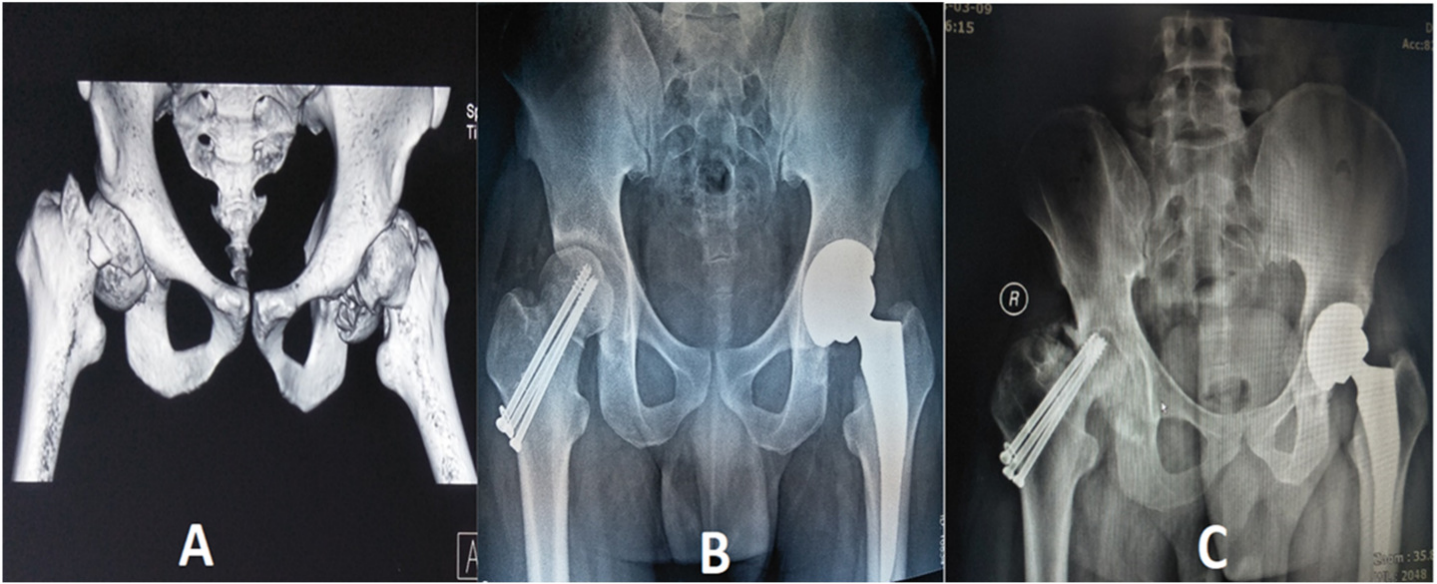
A 35-year-old man's (A) pre-operative 3D CT scan of the hip joint showed bilateral Pipkin III fracture-dislocation of the femoral head and neck. The right femoral head had two-part irregular fractures and a sub capital femoral neck fracture. The left hip had a comminuted femoral head fracture and subcapital neck fracture. (B) Postoperative radiographs revealing open reduction and internal fixation with three cannulated screws on the right hip and primary Total Hip Arthroplasty of the left hip. (C) Plain radiograph showing right-sided femoral head avascular necrosis with progressive shrinkage and collapse of the acetabulum dome within three years.
